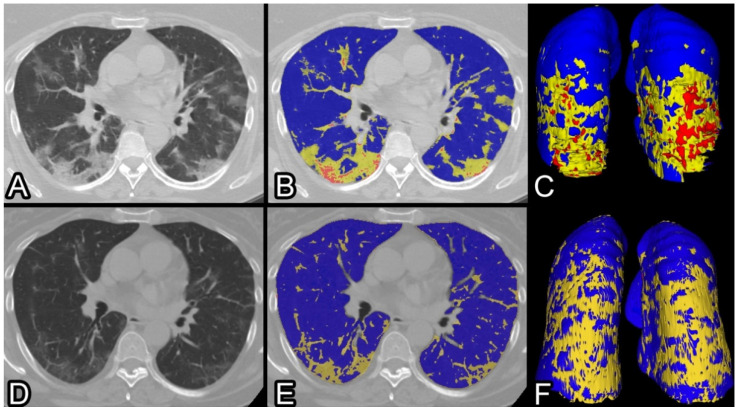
CT scans of a 57 years old lady affected by COVID-19 pneumonia, treated with non-invasive oxygenation during 11 days of hospitalization. At admission, she lamented dyspnea at rest, cough, thoracalgia and fever. (A) Non-contrast CT scan ad admission showing typical bilateral ground-glass consolidation (B) QCT of the same scan highlighting in yellow the poorly aerated and in red the non-aerated lung volumes (C) three-dimensional view of the whole lungs. After 44 days, a CT scan shows significant improvement (D) with subtle residual ground-glass opacities and linear scarring, better outlined at QCT (E) and using 3D reconstruction (F). At the follow-up clinical assessment, the patient lamented persisting laboured breathing and abrupt intermittent coughing. Residual %CL and %PAL were 6% and 5%, consequently %deltaCL and −%deltaPAL −12% and −10%.
